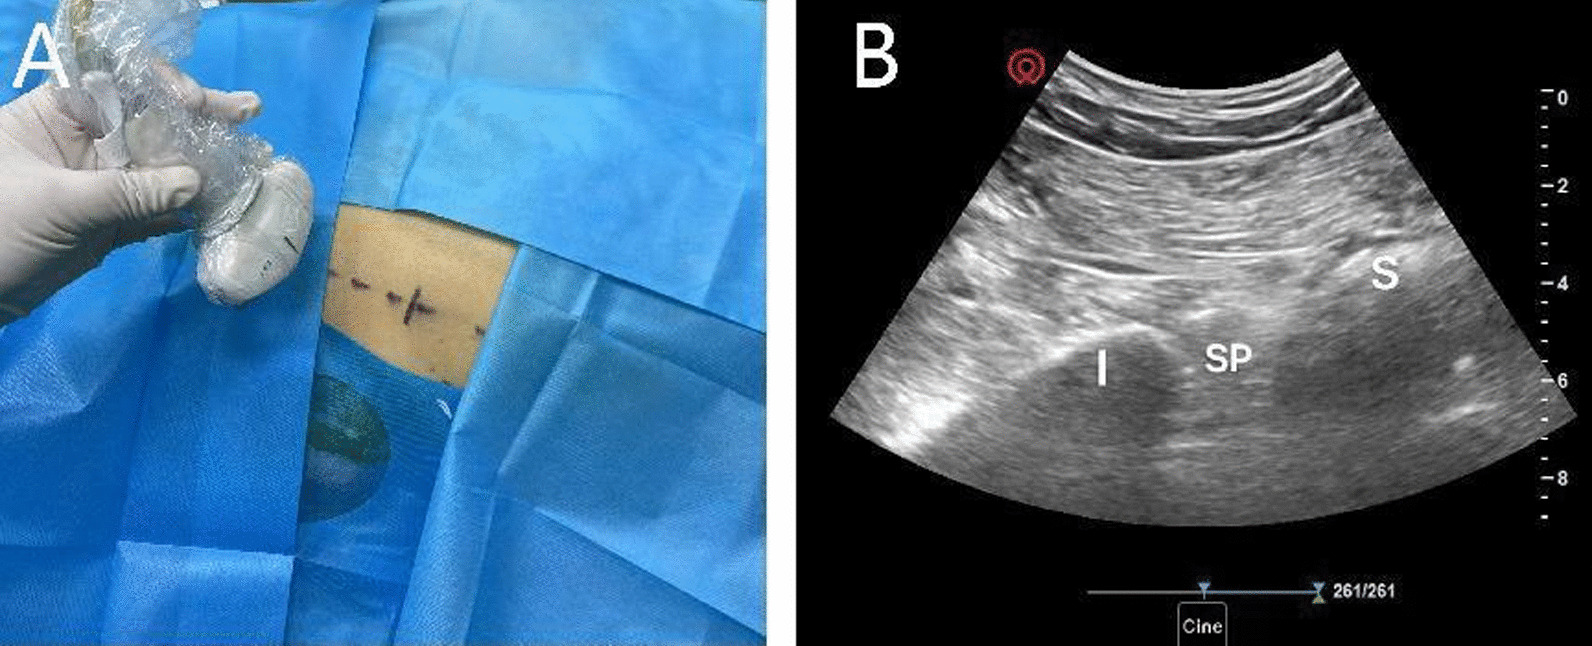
Approach of real-time ultrasound-guided sacral plexus block. *I* ilium, *SP* sacral plexus, *S* sacrum
